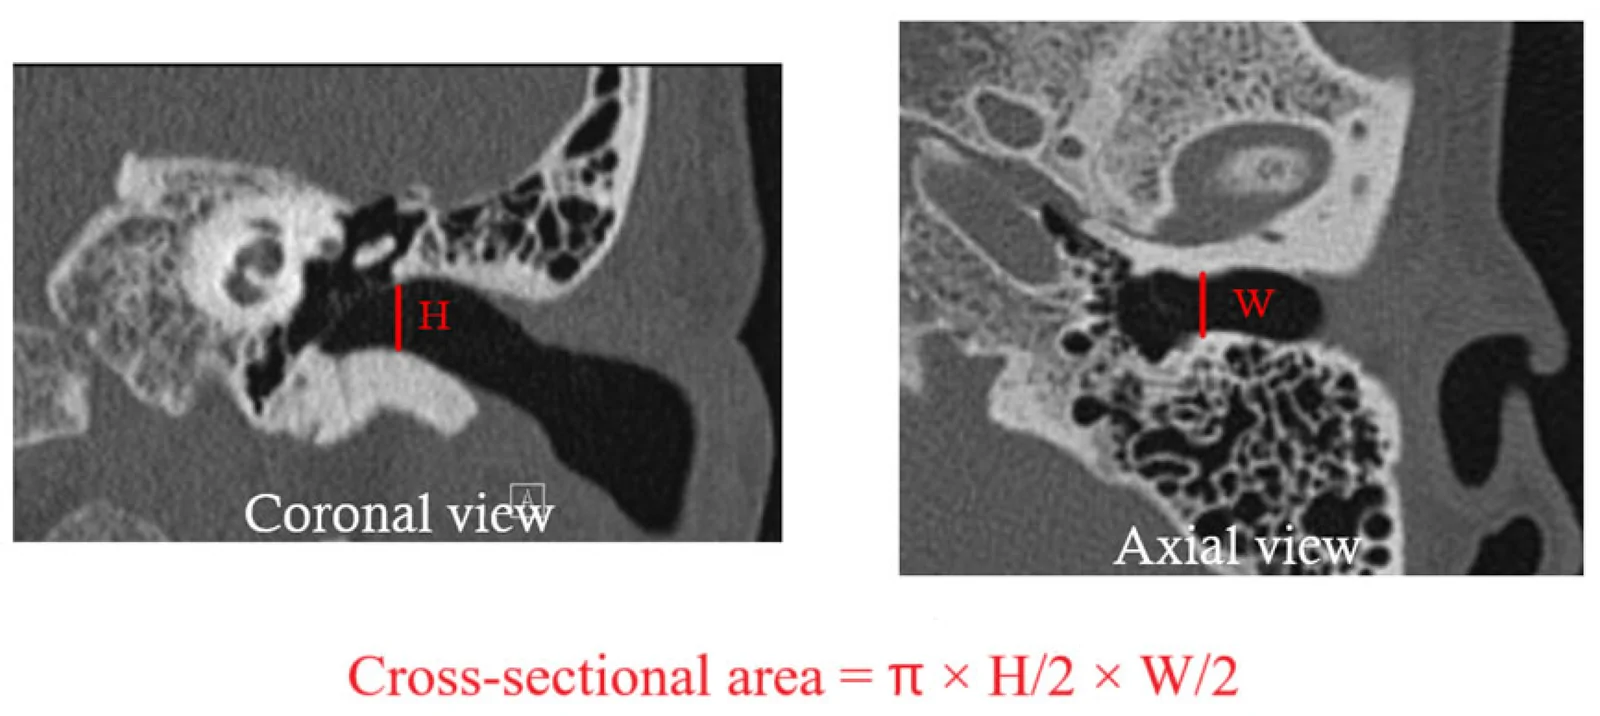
Measurement of the external auditory canal (EAC) isthmus dimensions on high-resolution temporal bone CT. The height (H) and width (W) of the EAC isthmus were used to calculate its cross-sectional area as follows: π × H/2 × W/2.
